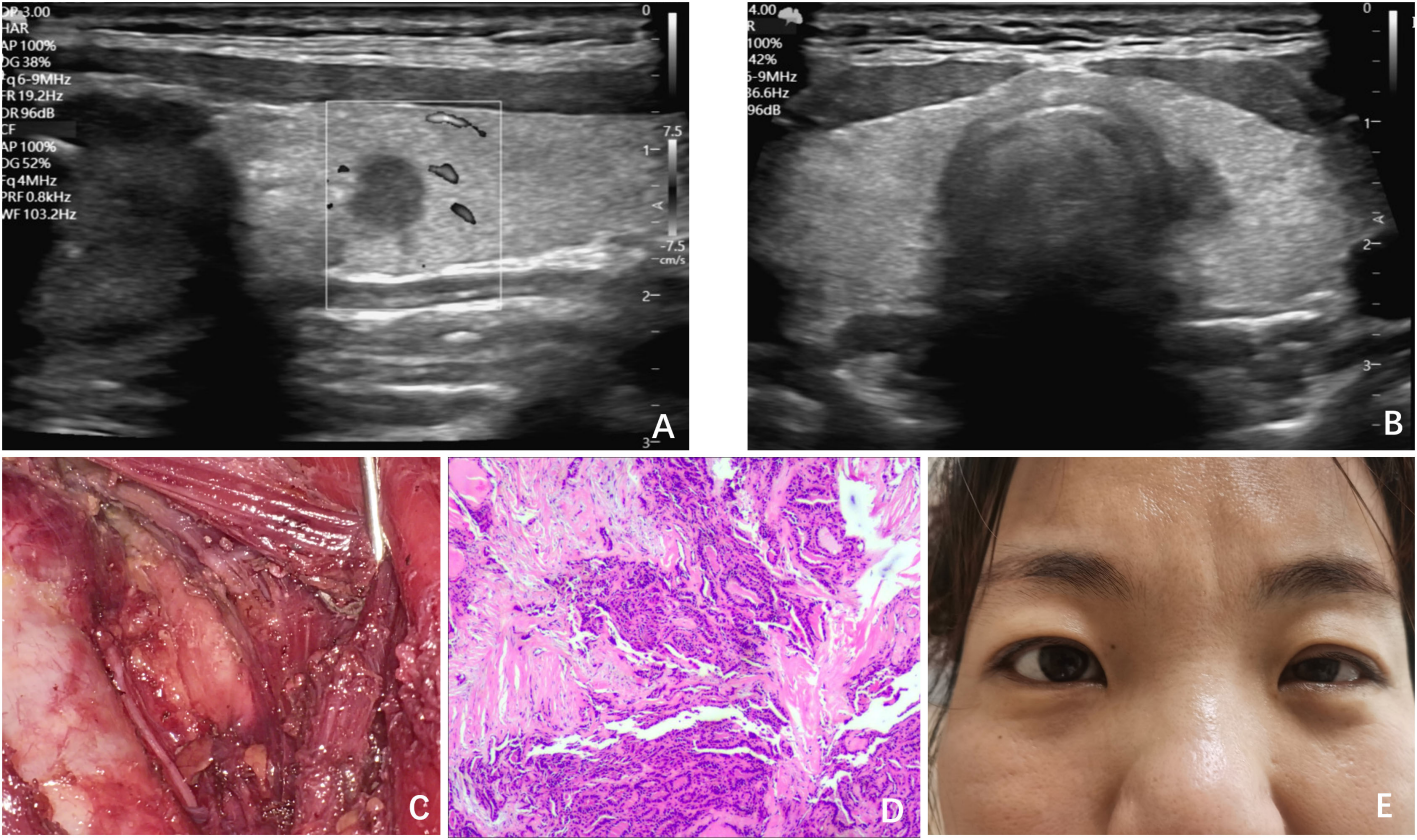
Clinical data of Case 2. (A) Ultrasound revealed a low echogenicity nodule, measuring 0.5 cm × 0.5 cm × 0.6 cm; (B) ultrasound revealed the nodule adjacent to the trachea in the left thyroid lobe; (C) surgical field after UT and CLND by ETS; (D) postoperative pathology indicated PTC; (E) on POD 1, ptosis of the left eyelid and miosis of the left eye was observed in the patient.
